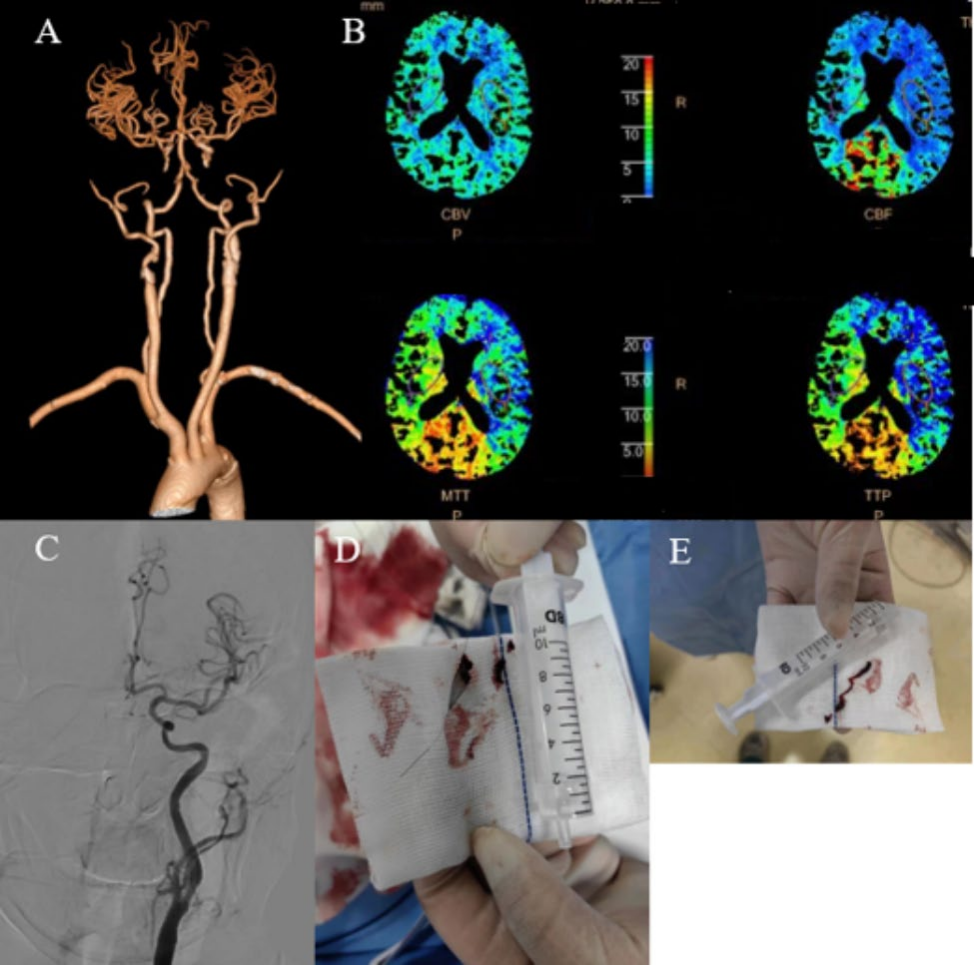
A shows the patient’s CTA after symptom aggravation. B shows the patient’s CTP after symptom aggravation. C shows DSA of the patient after thrombectomy. D-E shows the thrombus after thrombectomy
